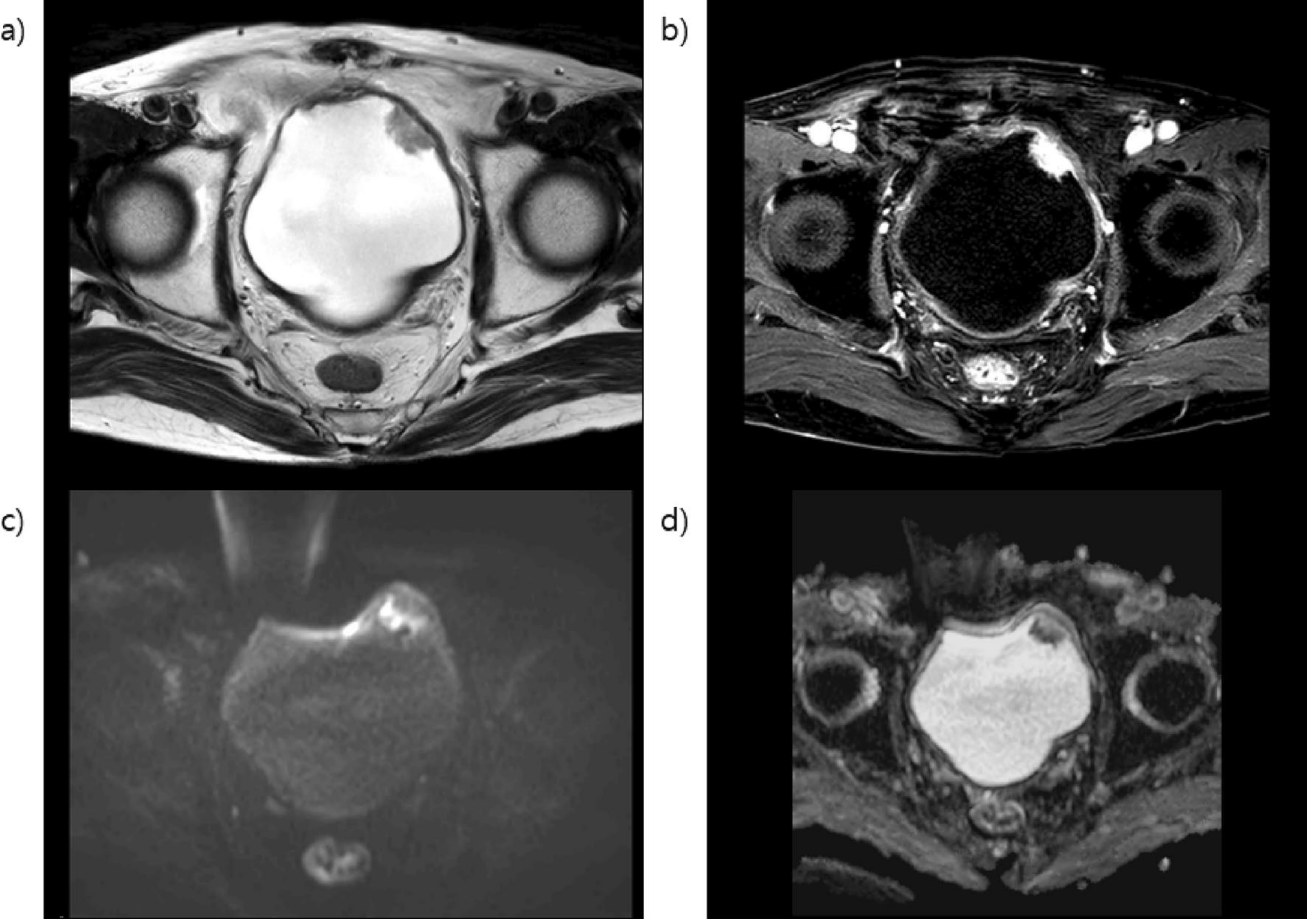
Magnetic resonance images of non-muscle-invasive bladder cancer (a) T2-weighted image in the left anterolateral aspect of the bladder wall showing a non-muscle-invasive papillary lesion preserving the hypointense detrusor lining underneath. (b) Dynamic contrast-enhanced imaging subtracted image displaying no early enhancement of muscularis propria. (c) Diffusion-weighted imaging (DWI) native image and (d) apparent diffusion coefficient (ADC) map showing tumor hyperintense on DWI and hypointense on ADC, with low SI thickened inner layer on DWI. The multiparametric magnetic resonance imaging patterns are consistent with non-muscle-invasive bladder cancer. *MRI* magnetic resonance imaging.
